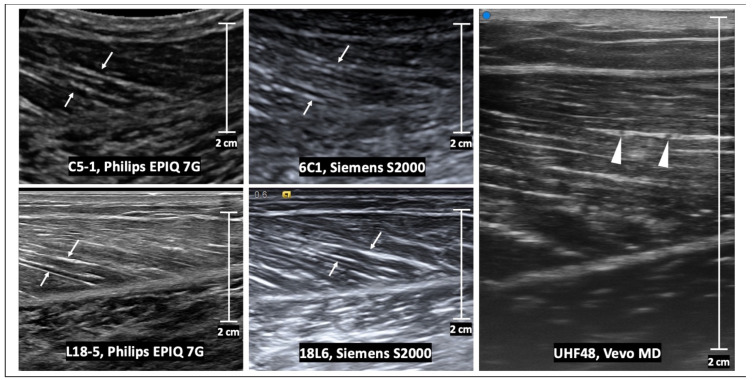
Transverse B-mode ultrasound images of the same normal muscle as in Figure 2. The internal muscle architecture is normal in all images, but the appearance differs with the transducer frequency as well as the vendor reconstruction algorithm. At low frequencies (e.g., using C5-1 and 6C1 transducers), there are subtle echogenic bands which represent the perimysium (arrows). At high frequencies (e.g., using L18-5 and 18L6 transducers), the echogenic perimysium is more apparent and the interfaces are better delineated. At ultra-high frequencies (e.g., using the UHF48 transducer), there is further improved resolution of the arborizing pattern of perimysium. Scale bars represent 2 cm.
